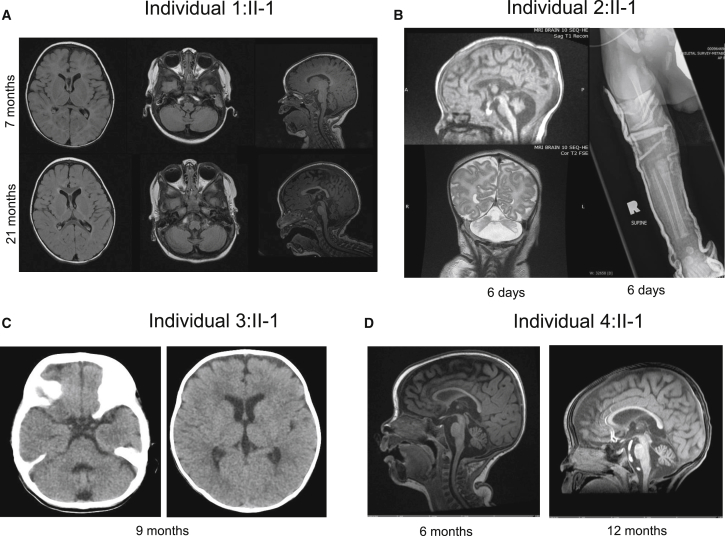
Radiographic Studies (A) Axial and sagittal T1-weighted images of the brain from affected individual 1:II-1 at 7 months (top) and 21 months (bottom) of age show moderate cerebellar predominant volume loss, which appears mildly progressive between the two exams, as well as mild cerebral atrophy with resultant enlargement of the ventricles. There is no brainstem atrophy. (B) Sagittal T1- and coronal T2-weighted images of the brain from affected individual 2:II-1 at 6 days of age show severe cerebellar and moderate cerebral and brainstem atrophy. A radiograph of the right leg also shows a mid-femoral fracture. (C) Two axial CT images of the brain from affected individual 3:II-1 at 9 months of age show mild prominence of the sulci and ventricles reflecting mild atrophy both above and below the tentorium. (D) Sagittal T1-weighted images of the brain from affected individual 4:II-1 at 6 months and 12 months of age show slightly progressive moderate cerebellar and cerebral atrophy. The brainstem is relatively spared.
